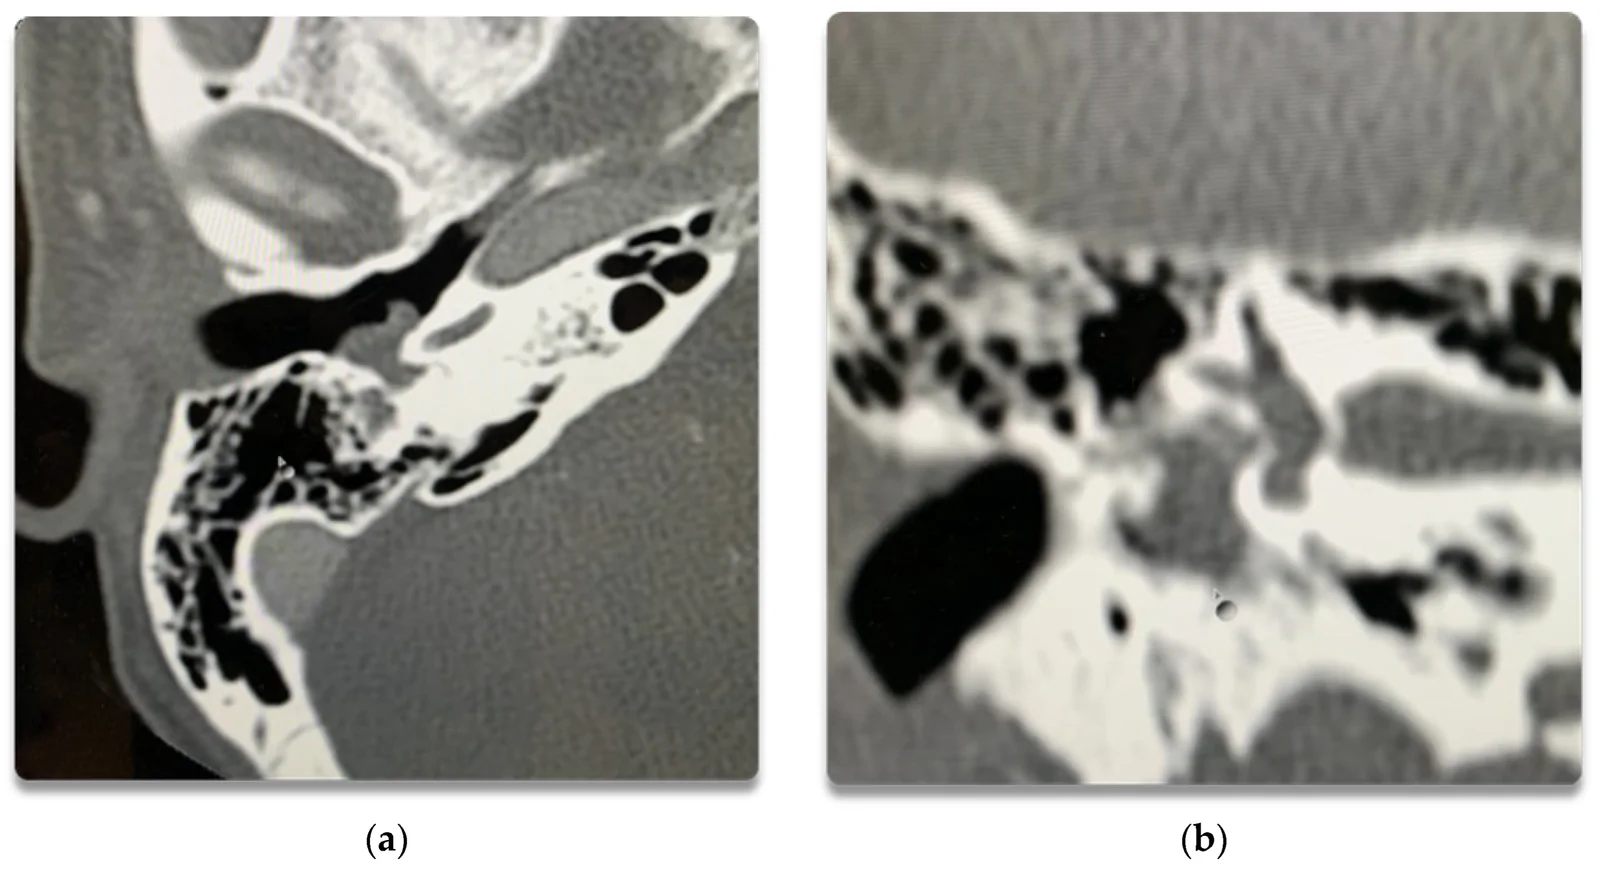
Temporal bone HRCT showing middle ear mass with extension to hypotympanum: (a) Axial view; (b) Coronal view.
